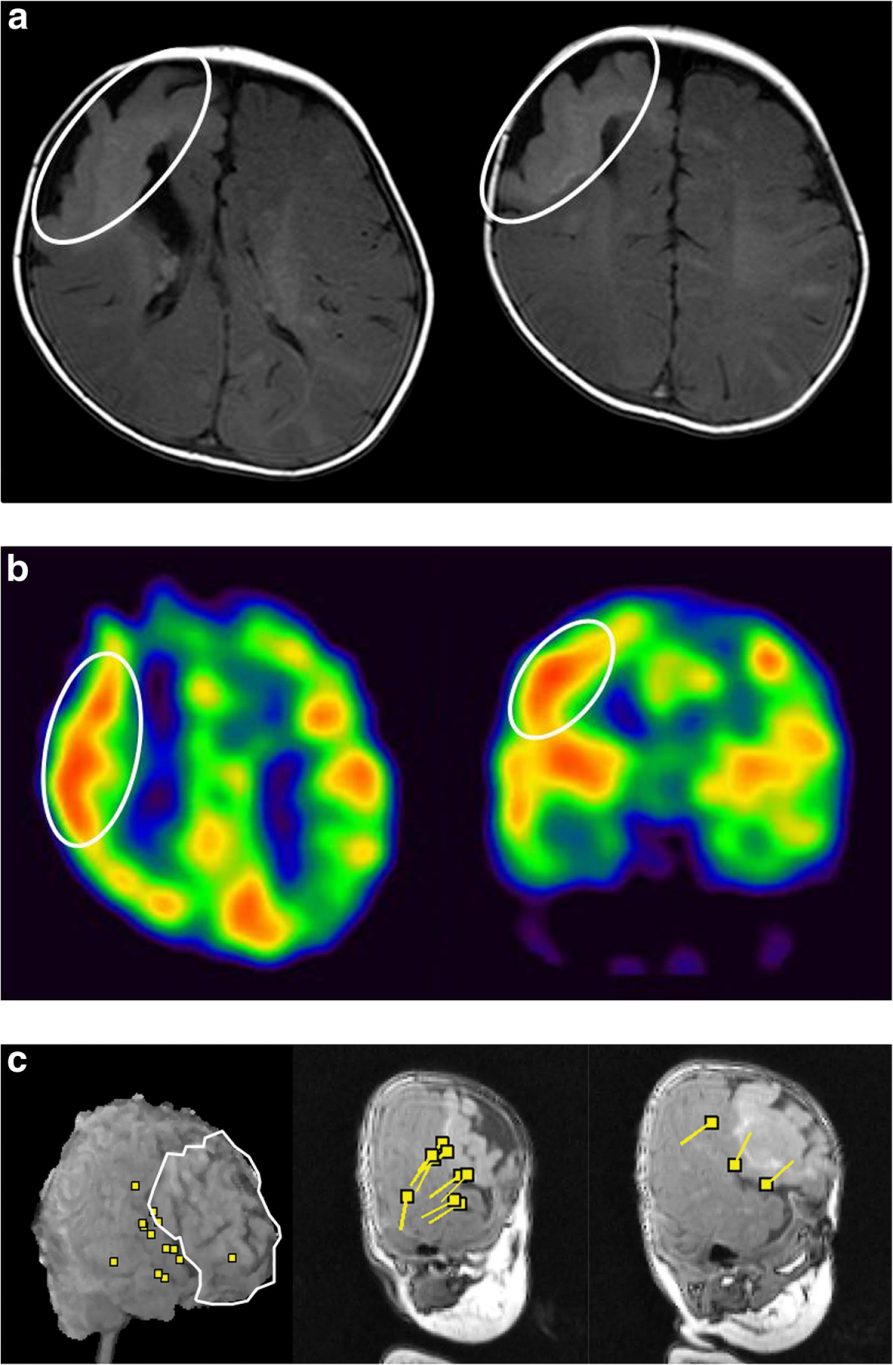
Magnetic resonance imaging, single-photon emission computed tomography, and magnetoencephalography at the age of 4 months. (a) Brain magnetic resonance imaging scan showing a slightly hyperintense lesion on T1-weighted images and cortical atrophy in the right frontal lobe involving the superior and medial frontal gyri and in the left parietal lobe. (b) Axial and coronal sections of ictal single-photon emission computed tomography scans. Increased perfusion was seen over the right central region (white encircled area). (c) A three-dimensional image and sagittal sections of the interictal magnetoencephalography scans. The region encircled by the white line indicates the cortical tuber. Spike dipoles were clustered at the pericortical tuber, and only one spike dipole was detected above the cortical tuber.
